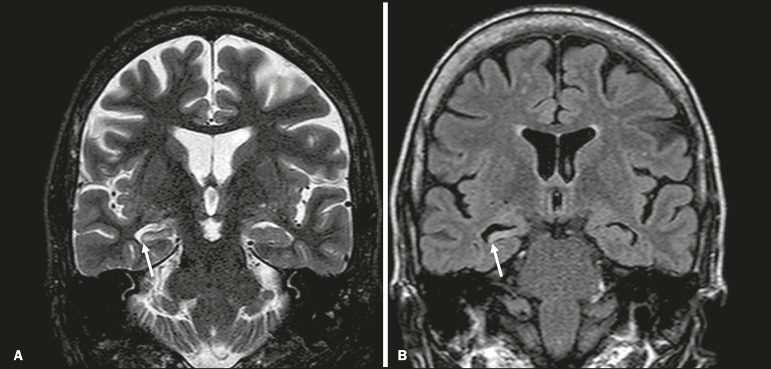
MRI of mesial temporal sclerosis. Coronal T2-weighted and FLAIR sequences (A and B, respectively), showing a hyperintense signal, atrophy, and right-sided loss of hippocampal digitations in a patient with mesial temporal sclerosis.
